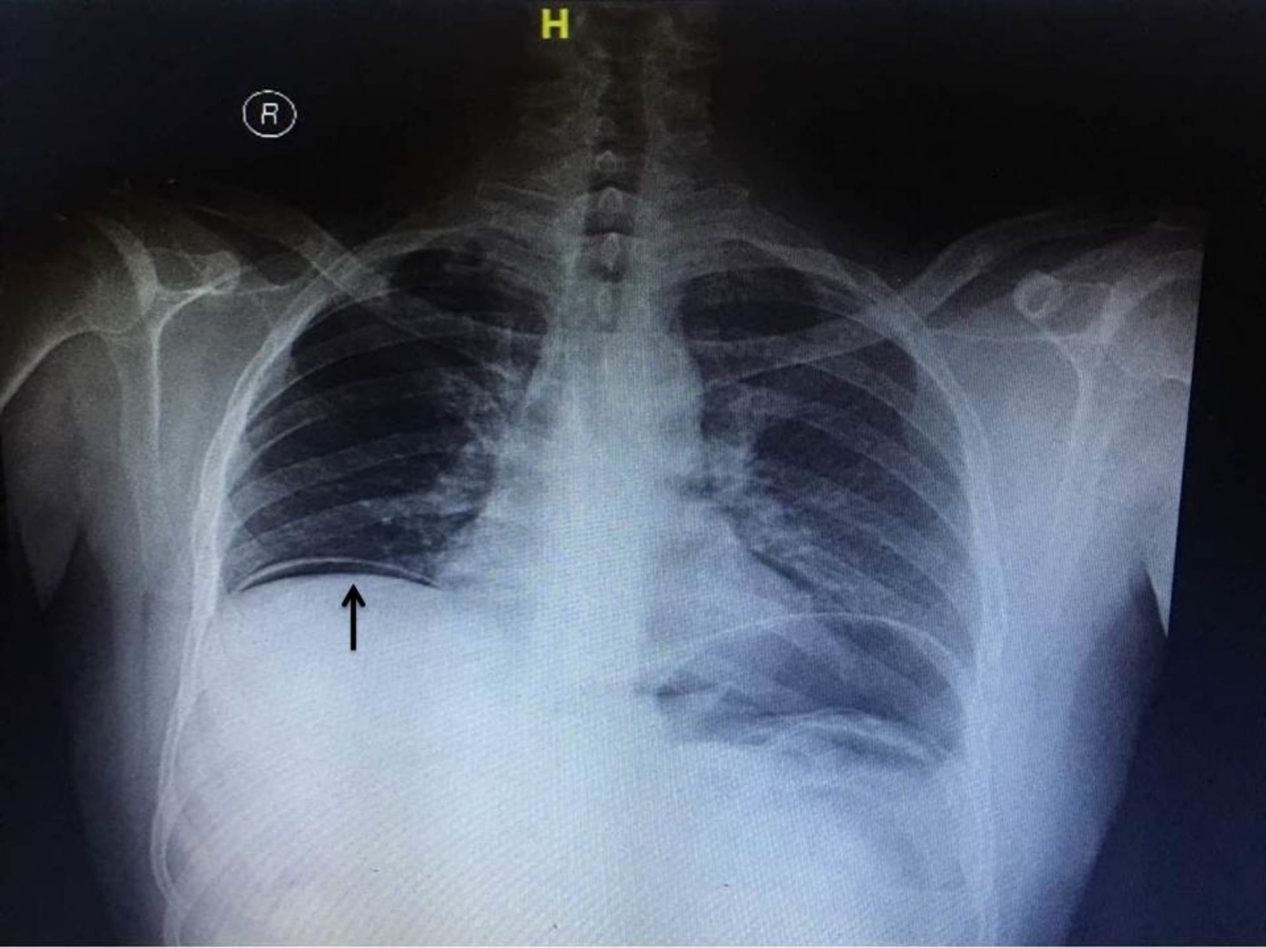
Preoperative x-ray with air under diaphragm (black arrow)
